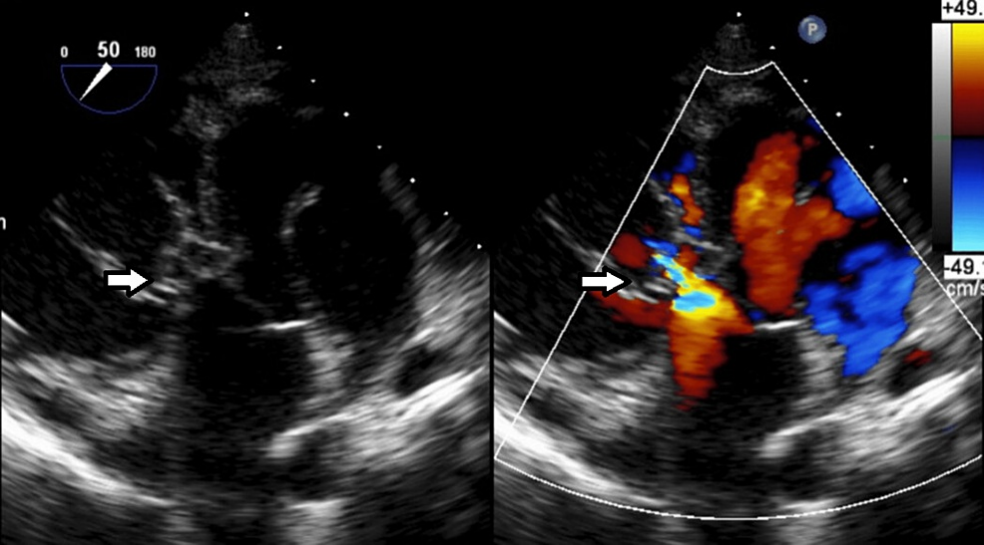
Transesophageal Echocardiogram (TEE). Deep transgastric TEE color-compare still frame showing a ruptured non-coronary sinus of Valsalva aneurysm into the right ventricle with left-to-right shunting (arrow).
